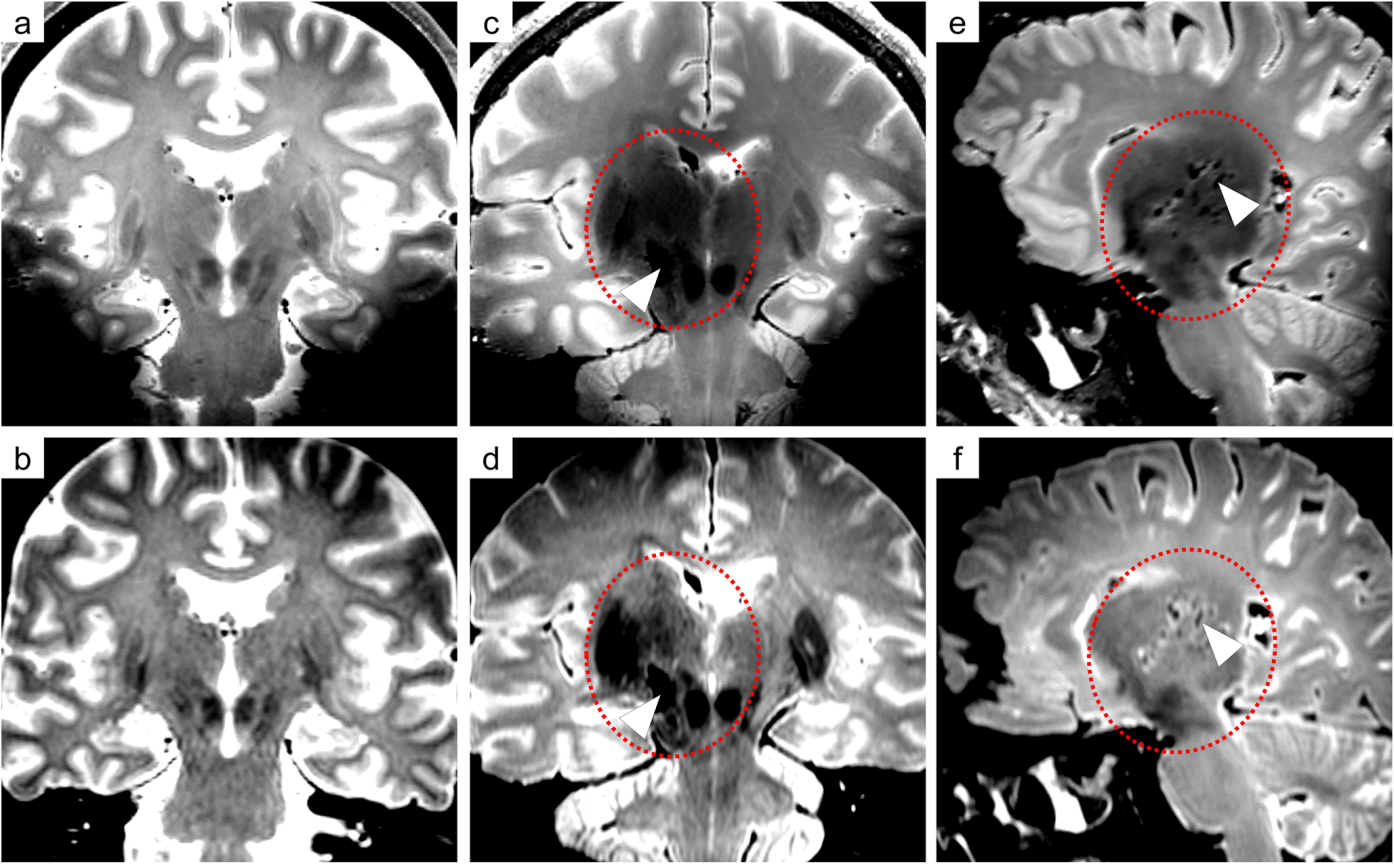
Comparison of the control case (a and b) with case 1 (c and d) and case 2 (e and f) in terms of the extent of cavitation injuries delineated by a dark/hypo intense zone (red circle) beyond the wound tract (arrowhead) on 7 T MRI (upper row) and on 3 T MRI (lower row). The dark/hypo intense zone was defined as damaged tissue. On 7 T MRI (c and e), the damaged tissue beyond the wound tract could be highlighted
